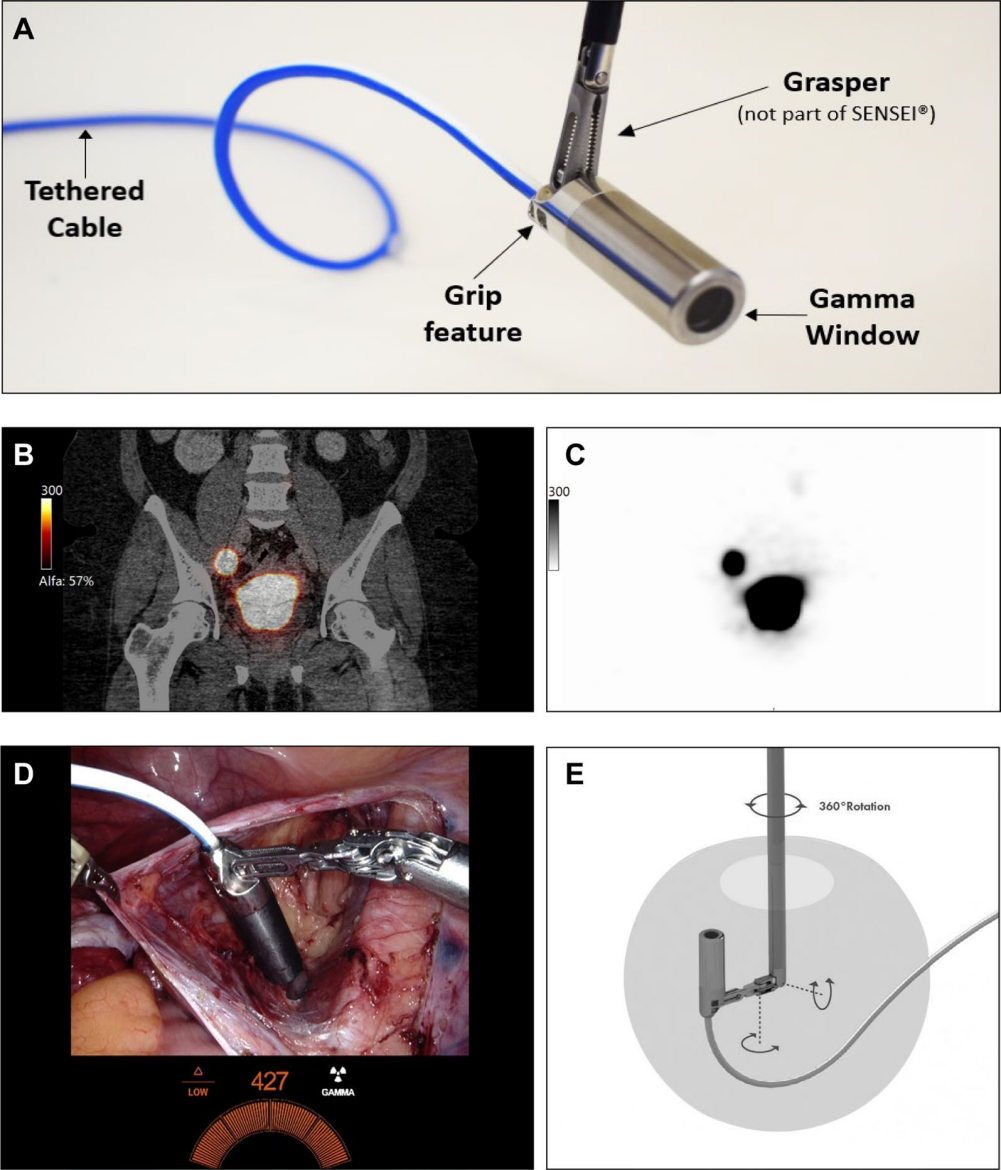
A Overview of the tethered drop-in γ-probe. B Coronal fusion SPECT/CT image at 90 min postinjection with 240 MBq ^99m^Tc into four quadrants of the cervix. The right SLN is localized in the obturator fossa. C Corresponding stand-alone SPECT/CT image. D Surgical view of robot-assisted radioguided SLN detection in the right obturator fossa. Integration of the gamma probe signal in the surgical view can be seen in the bottom center. E Rotational features of tethered drop-in γ-probe when grasped
